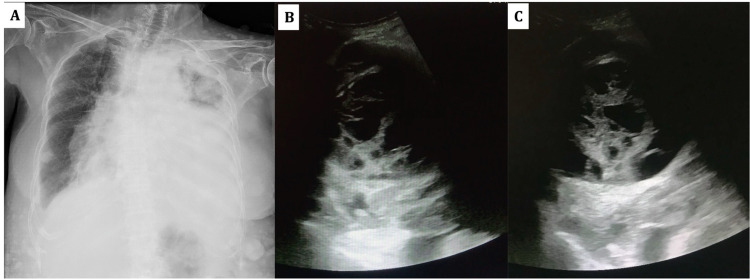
Chest radiograph and ultrasonographic image A - Chest radiograph showing left-sided pleural effusion with mediastinal shift to the opposite side and multiple subcutaneous neurofibromas; B, C - Ultrasonographic image of the left hemithorax showing effusion with multiple septations
